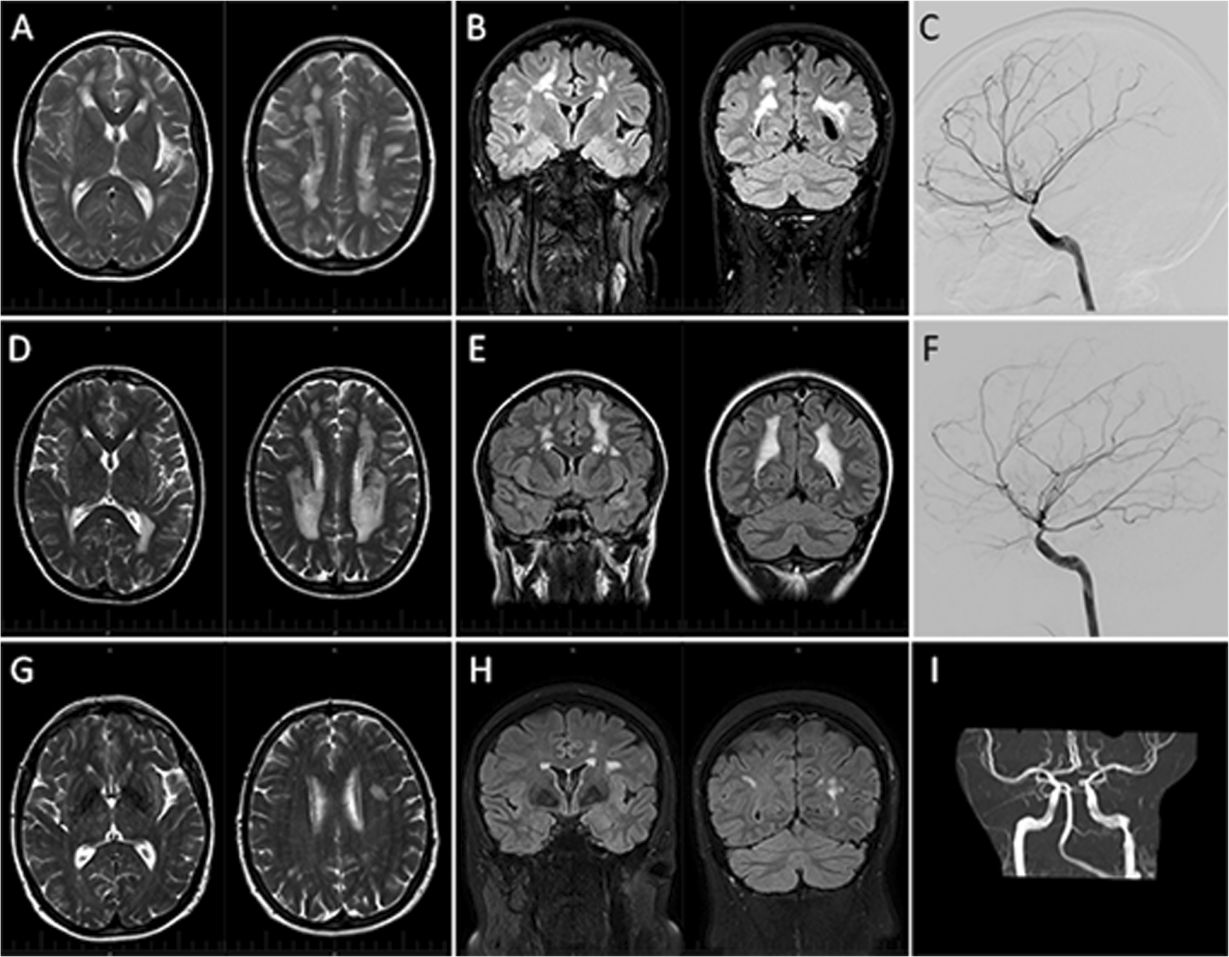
Proband’s axial magnetic resonance imaging at age 34 years showed extensive T2 hyperintensity in the periventricular and deep white matter of both cerebral hemispheres distributed predominantly in the deep water-shed regions whilst sparing the superficial water-shed territories (a). There was no sulcal FLAIR hyperintensity on coronal imaging (b). Catheter digital subtraction angiography (DSA) showed severe supraclinoid internal carotid stenosis, patulous petrous segment of the internal carotid artery, straightening of cerebral vessels and absent basal moyamoya collaterals (c). The proband’s older sister had similar distribution of T2 hyperintensity (d) and absence of sulcal FLAIR hyperintensity (e) at the age of 37 years. Her DSA showed similar a cerebral vascular anomaly that is distinct from moyamoya disease (f). The proband’s mother, aged 62 years, also had multiple foci of T2 hyperintensity in the deep and subcortical white matter of both hemispheres with physiologic calcification in the globus pallidi bilaterally (g). There was no sulcal FLAIR hyperintensity (h) and MR angiography showed fusiform dilatation of the internal carotid arteries within the proximal carotid canal bilaterally (i)
